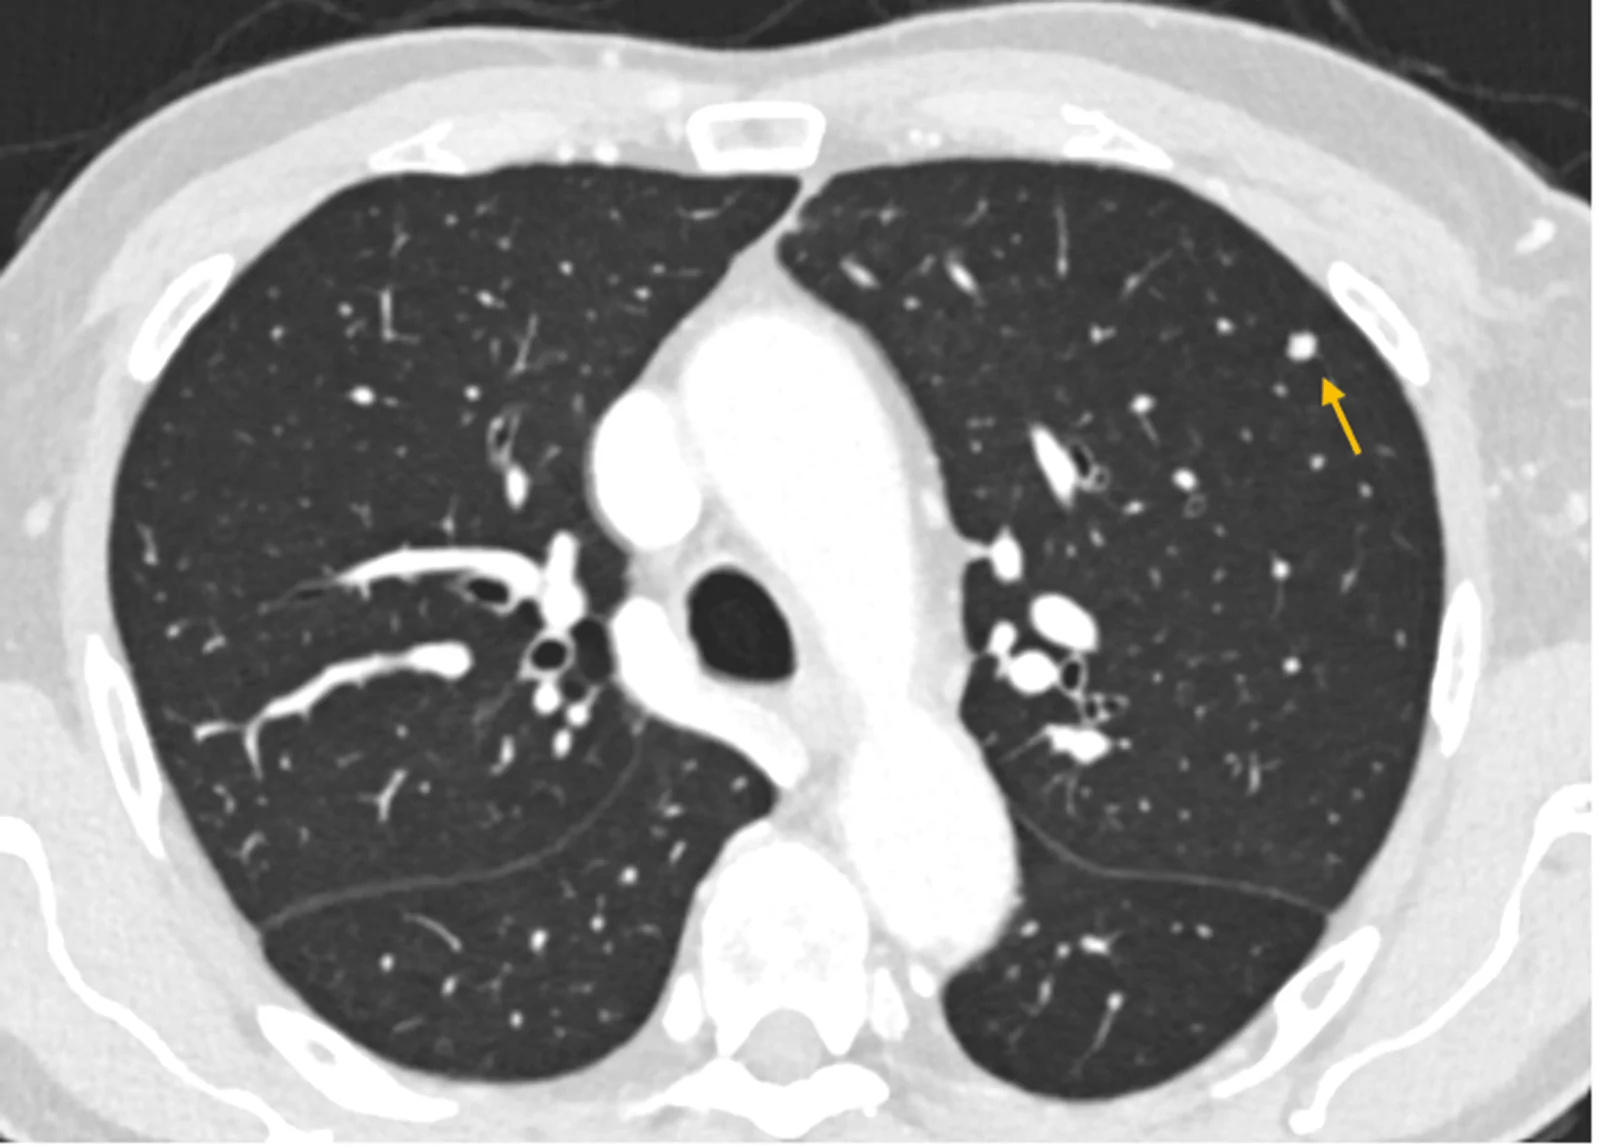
Contrast-enhanced axial CT image showing left lung metastases (yellow arrow).
